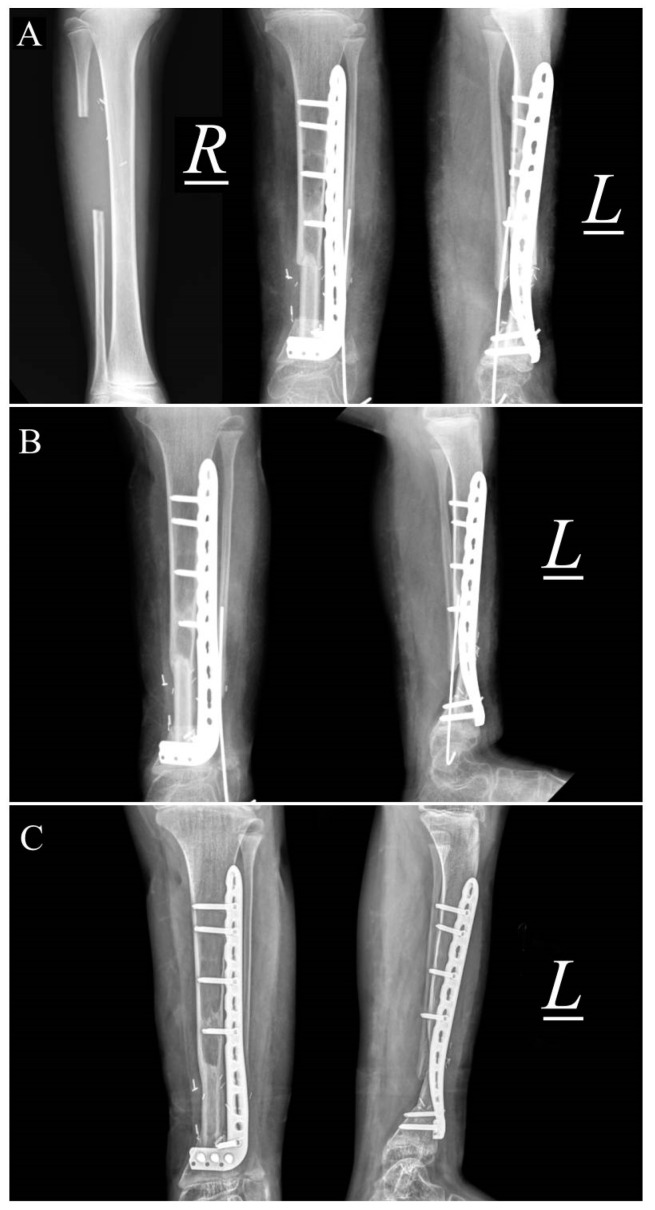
Postoperative follow-up X-ray images of patient 2: (A) postoperative 4 weeks with R indicating the right lower leg and L indicating the left. Both anteroposterior (AP) and lateral views are shown for the left. (B) AP and lateral X-ray of left lower leg at 3 months. (C) AP and lateral X-ray of left lower leg at 12 months.
